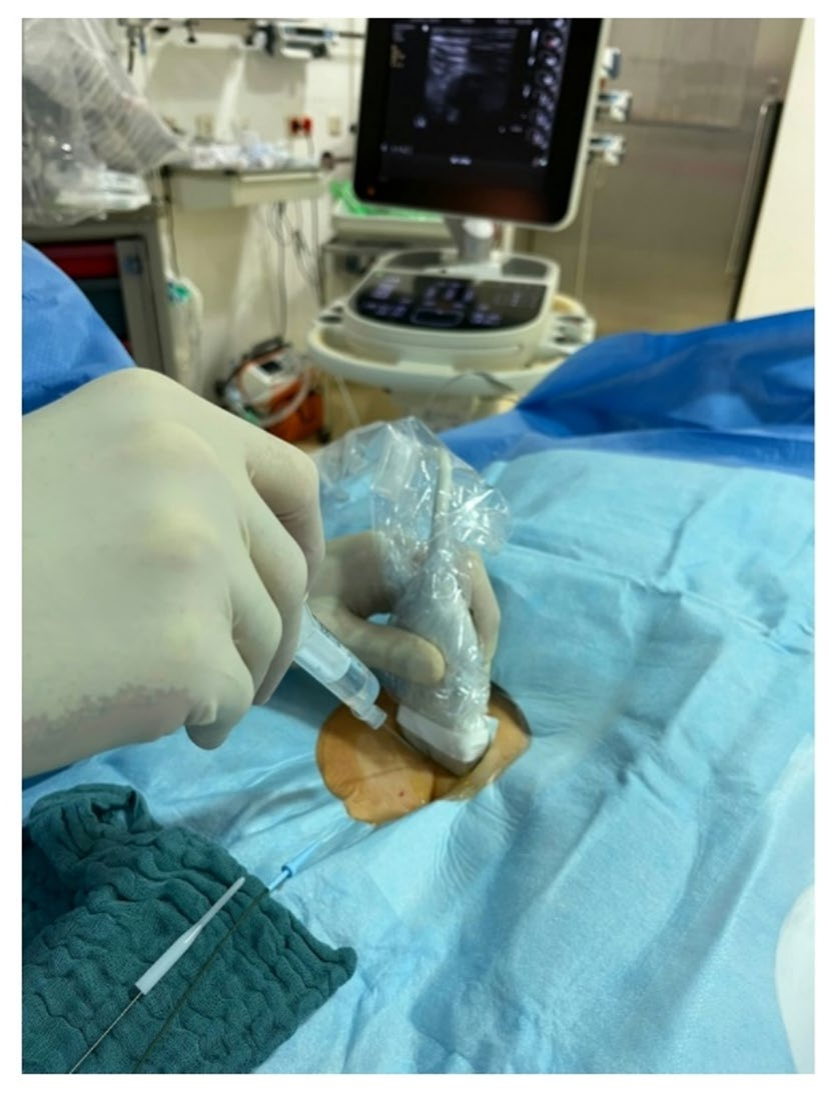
Venous access using ultrasound guidance via direct visualization. (Photo rights by J. Schipper MD).
